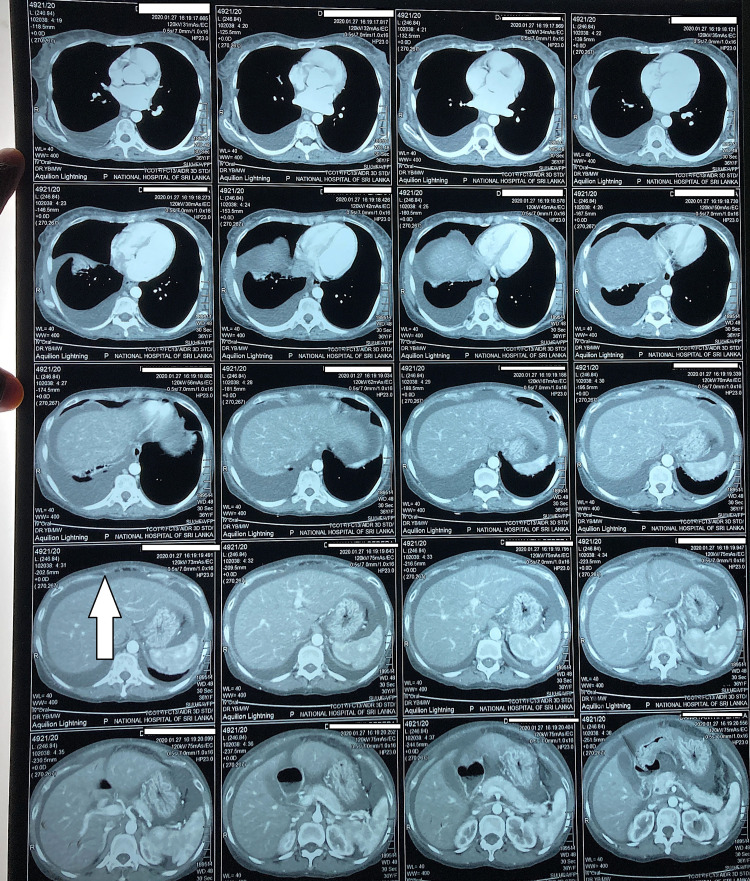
CT chest, abdomen and pelvis. Ascites fluid infront of liver.
